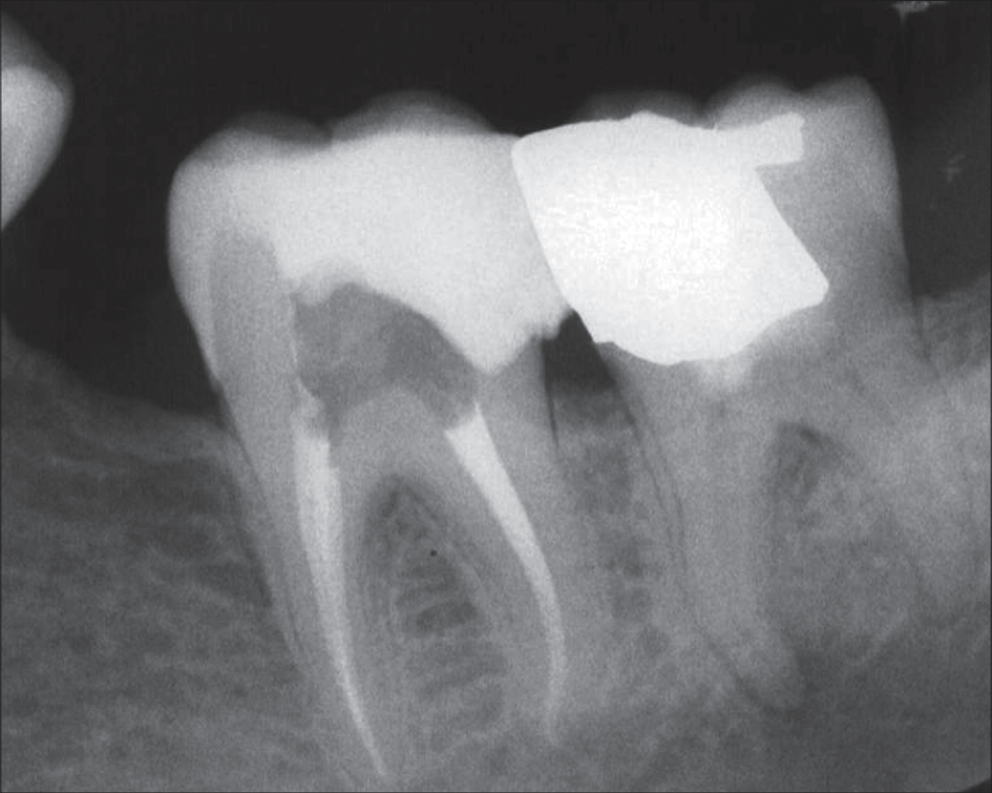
Immediate postoperative radiograph of the second molar after a week. Note the periradicular lesion in the mesial root of the third molar
